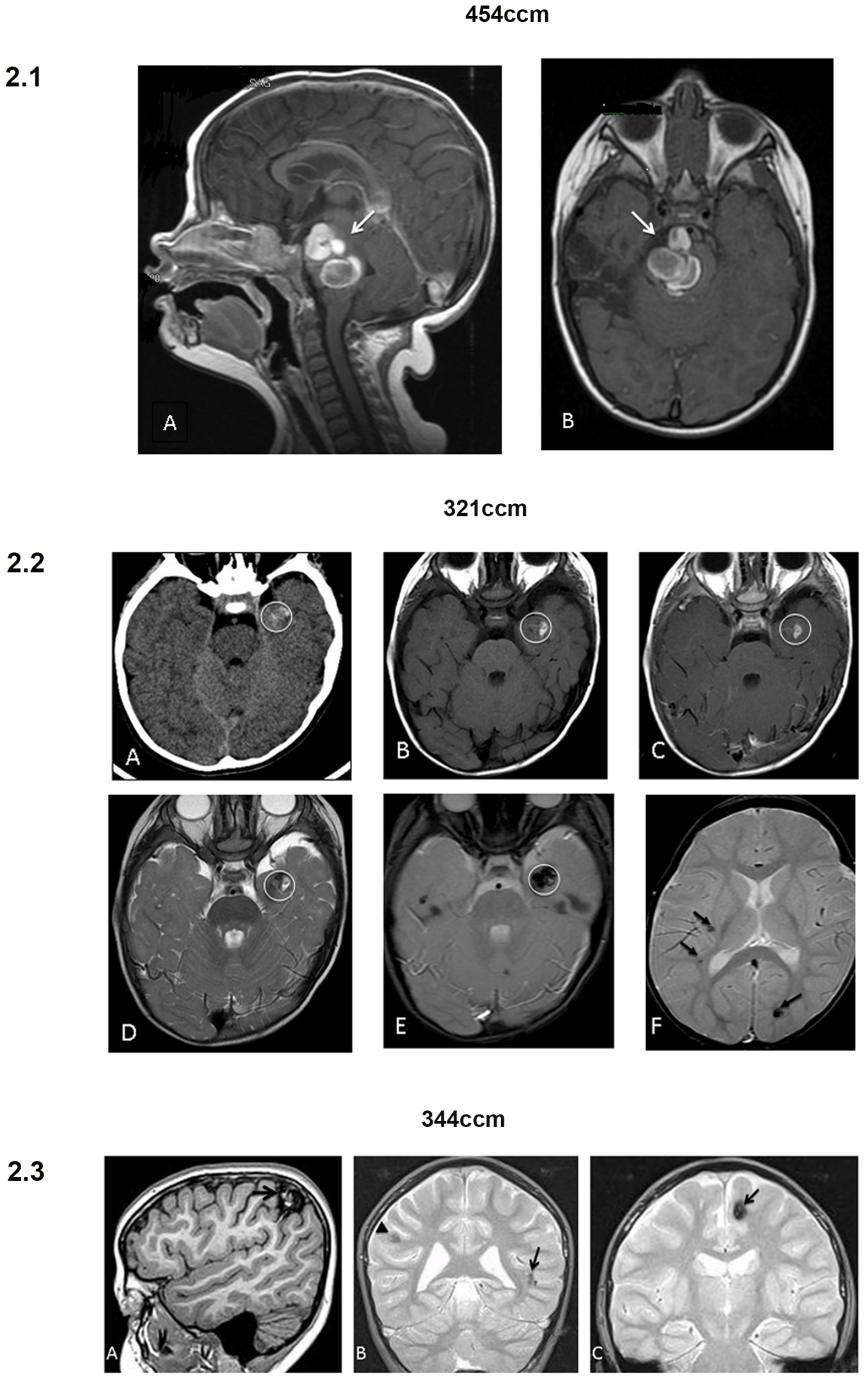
1–3 MRI/TC scans from subjects : 1) 454CCM patient harbouring the *de novo* and novel mutation p.E54Rfs*22. A) T1 sagittal image at 16 months showing a cavernous malformation with recent bleeding in the pons; B) T1 axial image at 25 months showing increased size of the pontine cavernous malformation with compression on the mesencephalon, the cisterna interpeduncularis and the cisterna pontis. 2) 321CCM patient harbouring the mutation p.R35X. A) Imaging characteristics of the CCM lesion (in the white circle) located at the left anterior temporal lobe: at CT scan the lesion is inhomogeneous due to haemorrhagic components, B and D) the haemorrhagic component is hyperintense both in T1 and in T2 sequences, C) contrast enhancement is absent, E) and at GET2* the lesion is hypointense due to the paramagnetic characteristics of the haemosiderin ring and of the clotted lesion content. F) Finally, other two lesions can be detected at other sites (arrows) in the same patient. 3) 344CCM patient harbouring the novel mutation p.R54X. A) MRI showed a right cortical and subcortical parietal hemorrhagic CCM lesion (arrow) and other non-hemorrhagic CCM lesions at different sites: bilateral temporal polar (not shown), B) left superior temporal sulcus (arrow) and right parietal (arrowhead), left insular and fronto-insular (not shown), C) left frontal parasagittal (arrow), subcortical frontal with small areas of vacuolization and microcalcification, left posteromedial thalamic (not shown).
